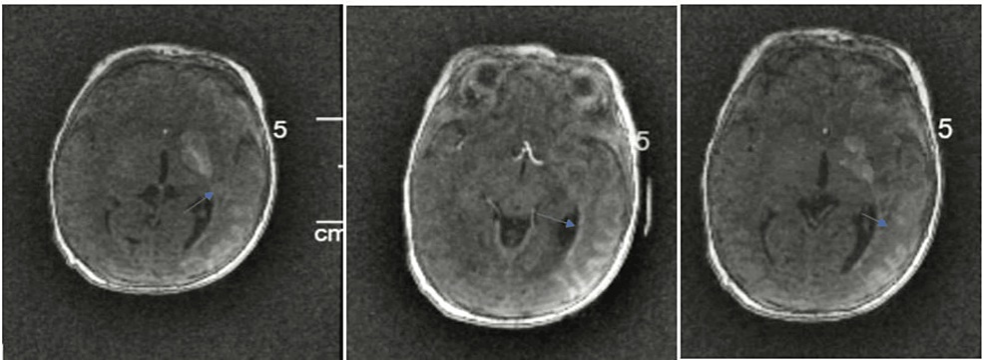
MRI and MRA findings MRI and MRA brain demonstrated a large infarct involving the entire territory of the left middle cerebral artery, involving the left frontal, temporal, and parietal lobes, insula, and basal ganglia that is being shown by the arrows. A much smaller infarct is demonstrated in the right MCA territory in the right temporal lobe and small area of the right parietal lobe. T2 hyperintense edema and swelling are demonstrated throughout the left MCA territory. No midline shifts. Ventricles are normal in size.
